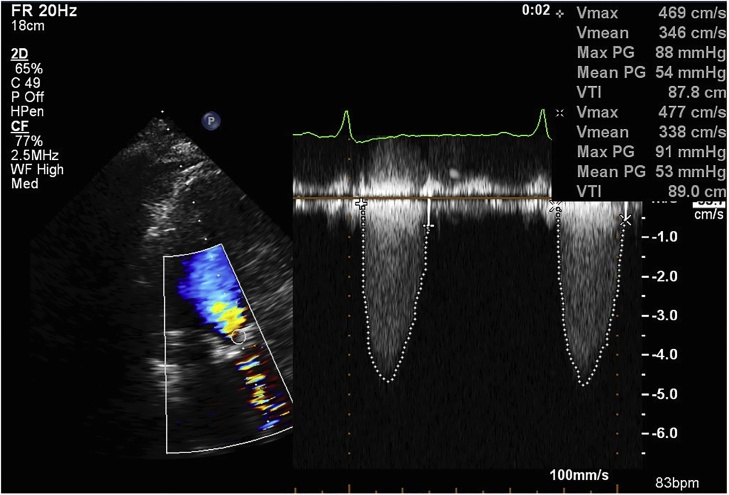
Preoperative echocardiography showing severe aortic stenosis.
